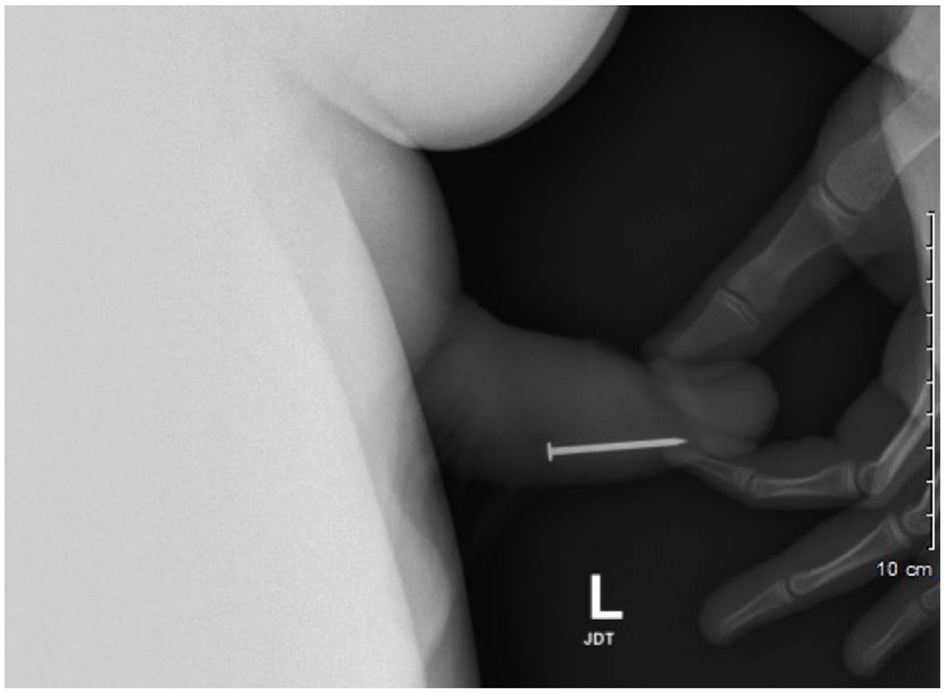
Lateral view pelvic X-ray identifies nail in distal penile urethra, which requires endoscopic removal.
